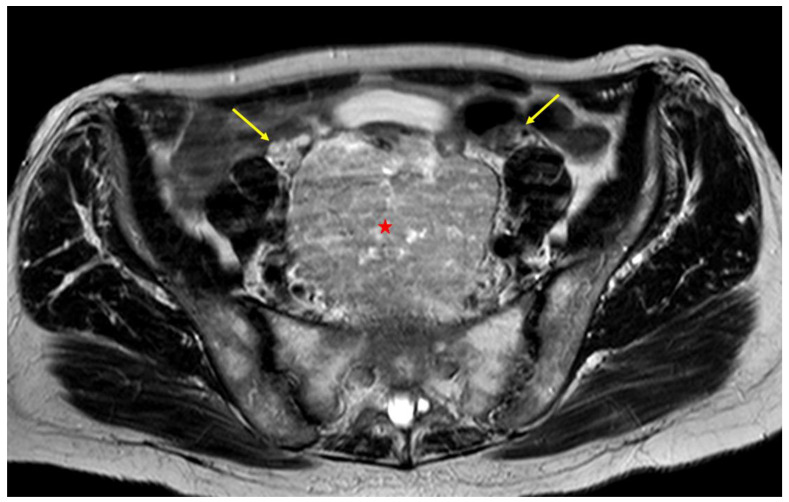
Our case: T2 axial MRI image depicting normal ovaries (yellow arrows) and GIST (red star).
